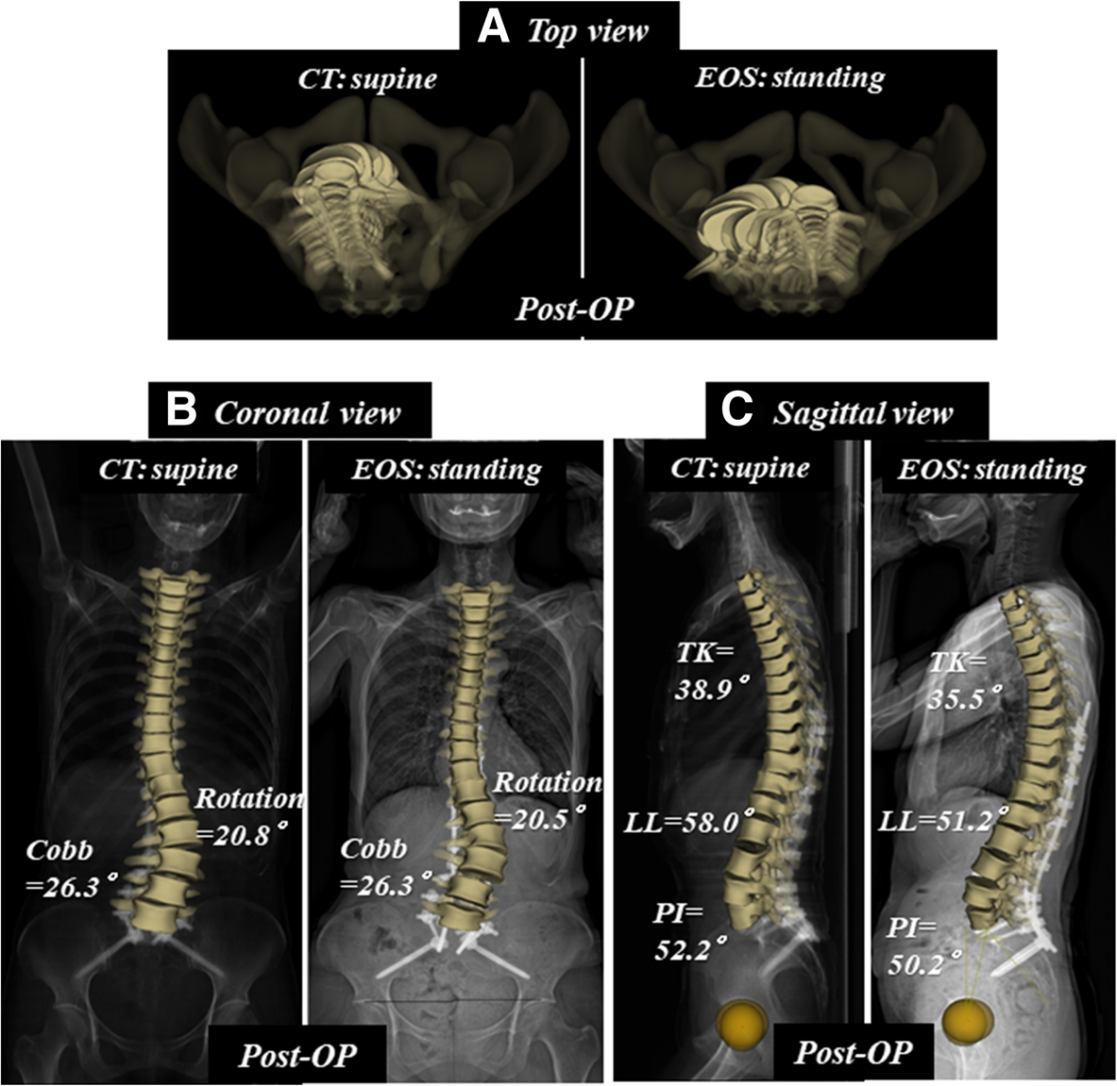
Patient with degenerative kyphoscoliosis (Fig. 2) treated by posterior correction and fusion from T9 to pelvis. Postoperative supine CT and standing EOS images. a Top view images. b Coronal images. c Sagittal images
